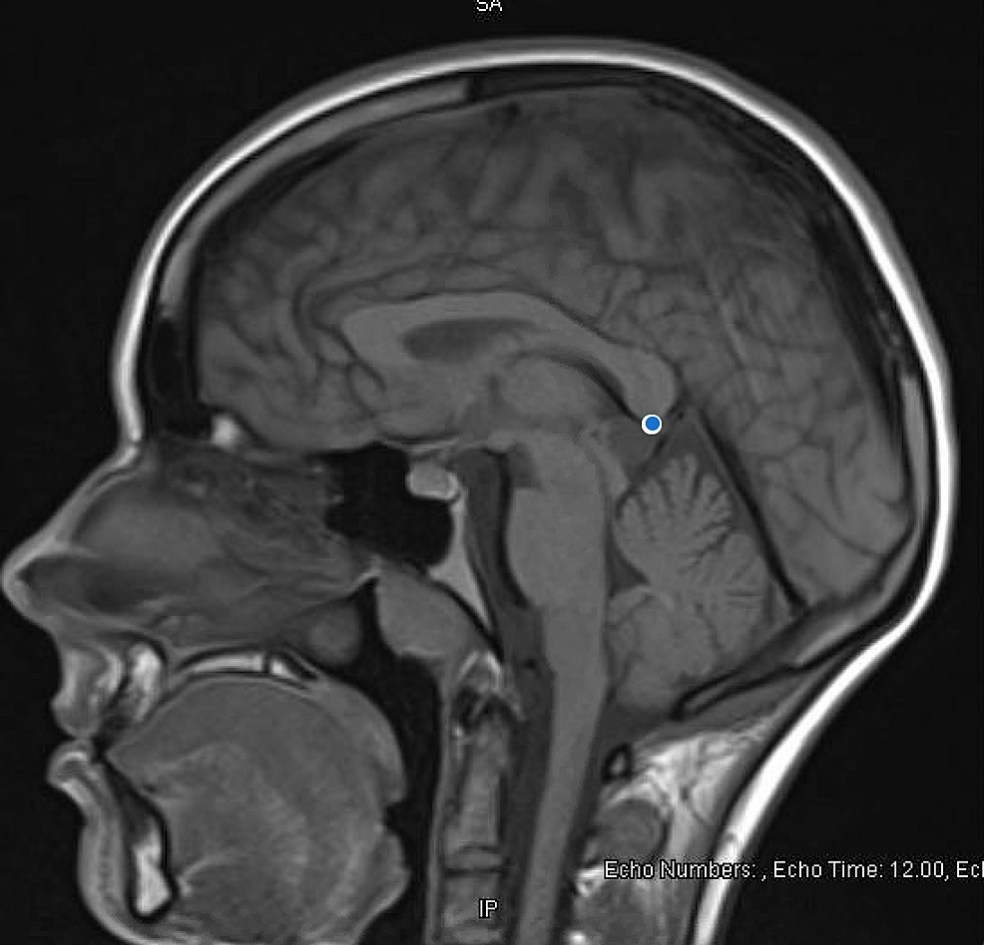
MRI of the brain without contrast sagittal view showing pineal gland cyst Radiologic report:* lesion within the region of the pineal gland, which is T1 hypointense and T2 hyperintense, measuring 1.3 x 1.3 cm, likely consistent with a pineal cyst.* A blue dot marks the area of interest.
